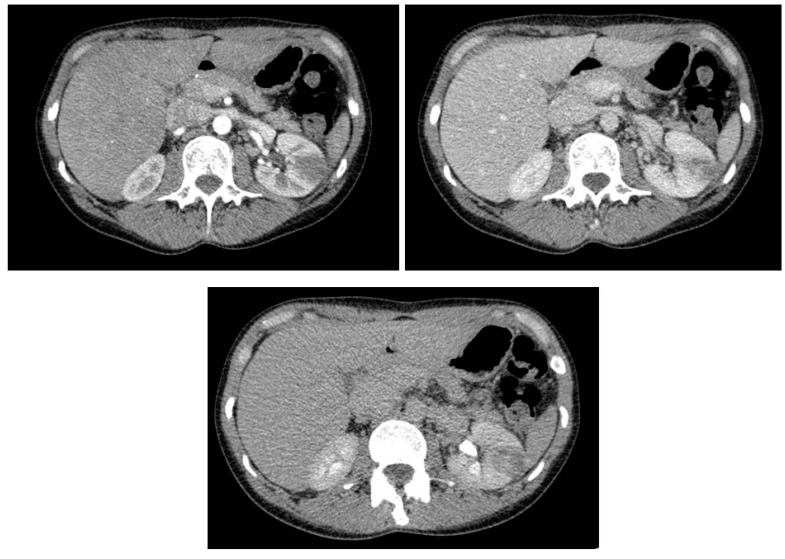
Abdomen CT-scan with contrast medium. The left kidney is slightly larger in size than the contralateral one, and it presents a large cortical area of reduced post-contrast impregnation in the middle with a maximum axial diameter of approximately 2.8 cm. The findings are referable to acute pyelonephritis.
